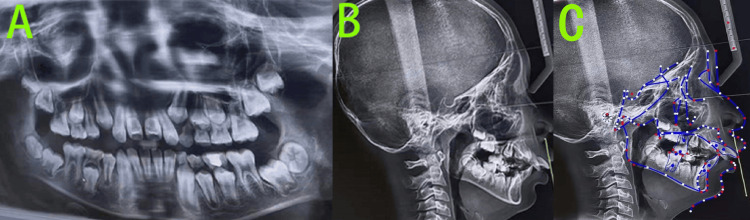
Pre-treatment radiographs A: Panoramic radiograph revealed the number of permanent tooth buds was within normal limits; B: Lateral cephalogram; C: Cephalometric tracing indicated a skeletal Class II pattern with mandibular retrognathia, a high mandibular plane angle, an average growth pattern, severely proclined upper incisors, and labially inclined lower incisors. Cephalometric tracing in Figure 2C created using the UCeph software (version 3.1, UCeph Software, Chengdu, China).
